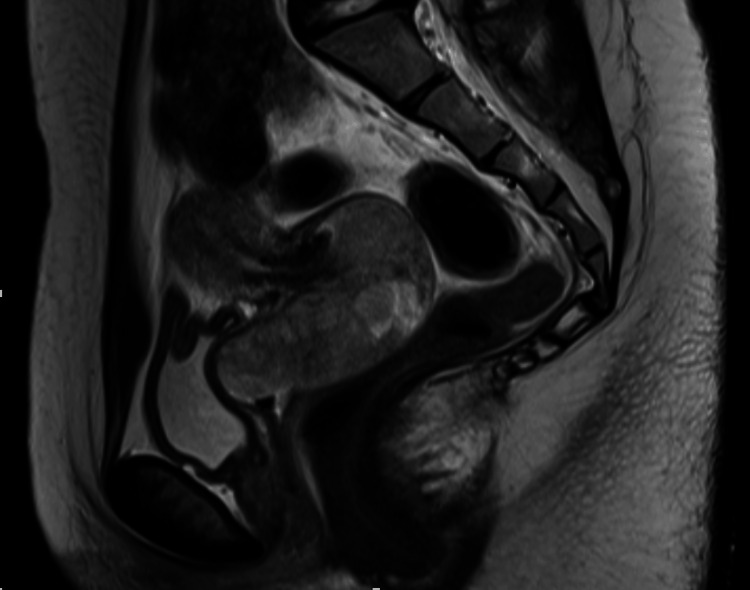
The representative image of MRI Contrast-enhanced T2-weighted MRI reveals a 68 × 37 mm mass originating from the uterine cervix and protruding into the vagina. No evidence of bladder or rectal involvement is observed.
